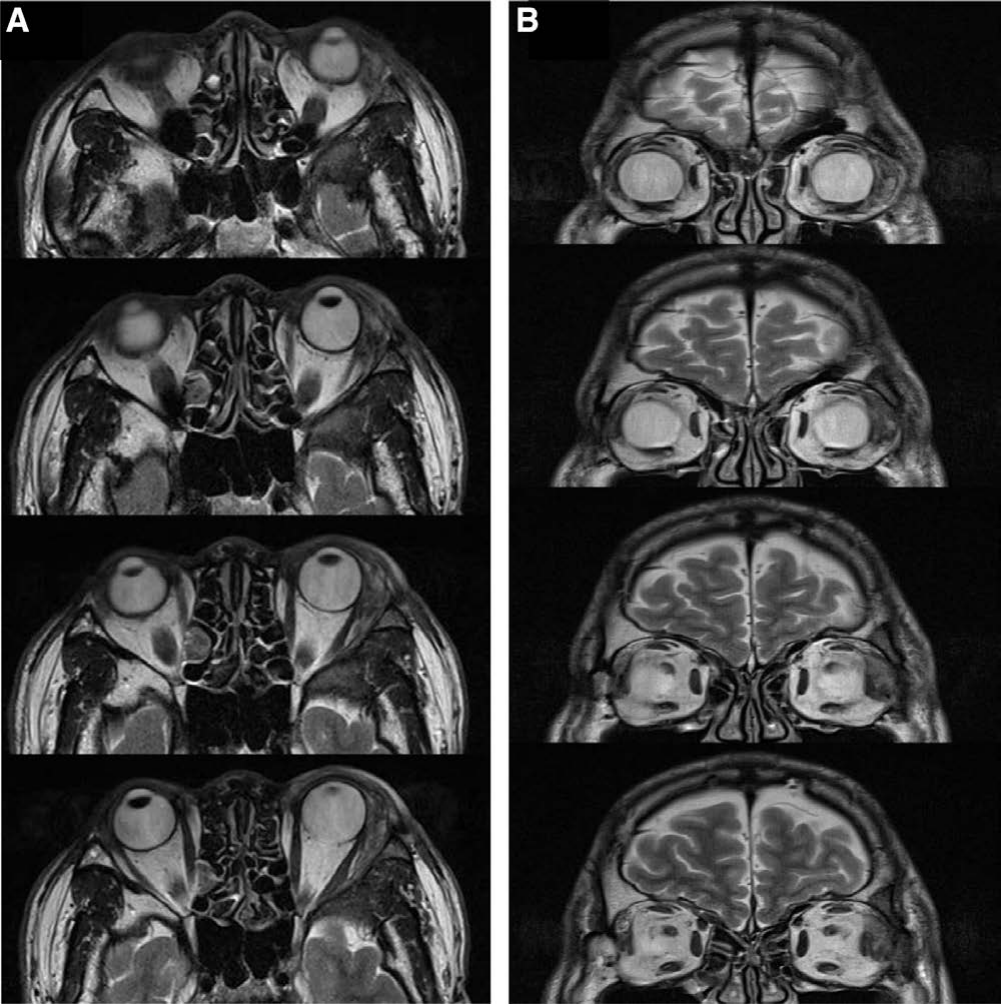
T2-weighted axial, (A) and coronal (B) magnetic resonance image showing left orbital mass in the area of the lacrimal gland (bony wall of orbit was infiltrated, the periosteum was intact).
